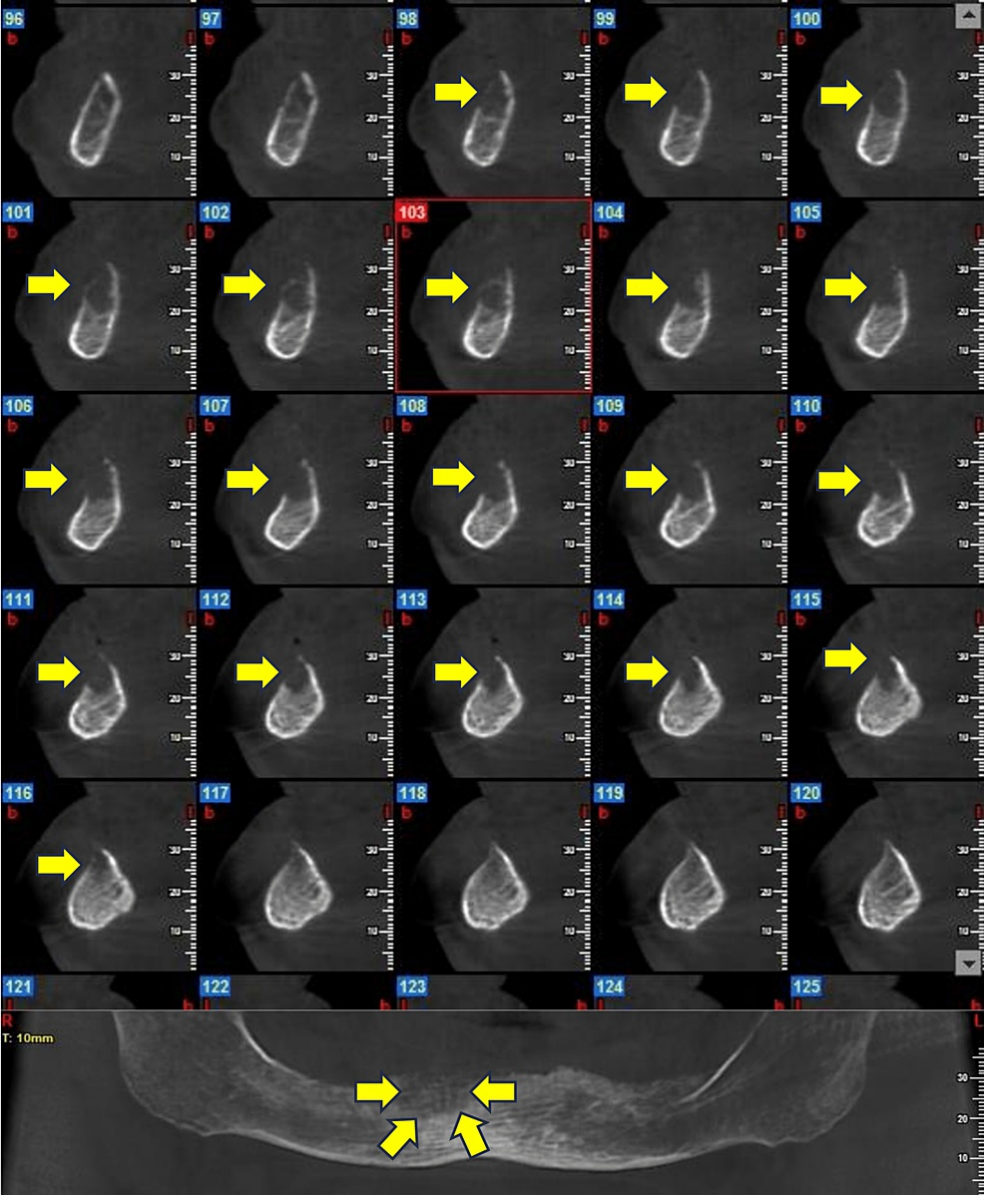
Three-dimensional radiographical imaging of the osteolytic lesion in the fourth quadrant (yellow arrows indicate the radiolucent lesion)
